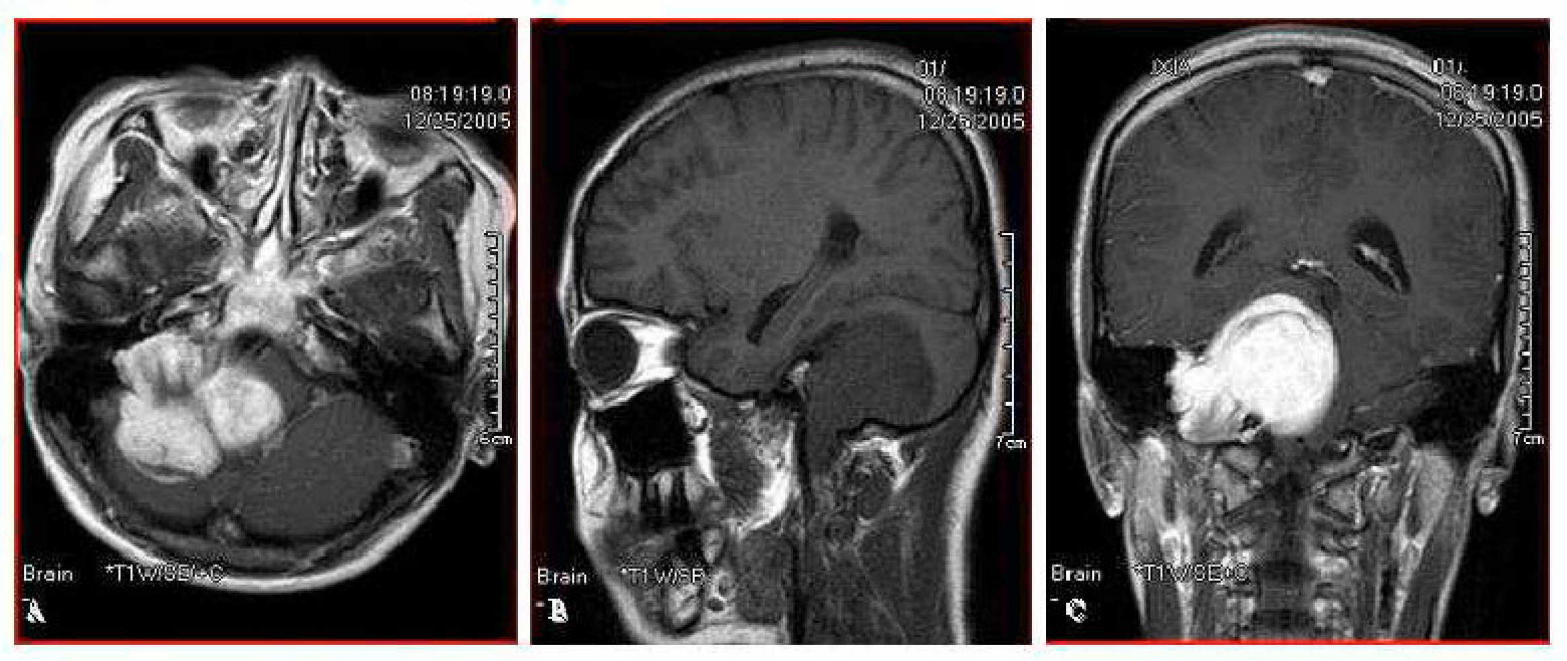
(A) Axial gadolinium-enhanced T1-WI magnetic resonance image shows an enhanced lesion in the right cerebellopontine angle, with compression and distortion of the brain stem and cerebellum. (B and C) Sagittal T1-WI (B) and coronal gadolinium-enhanced T1-WI magnetic resonance images (C) display a significantly enhanced mass in the right cerebellopontine angle with an extracranial extension through the jugular foramen.
